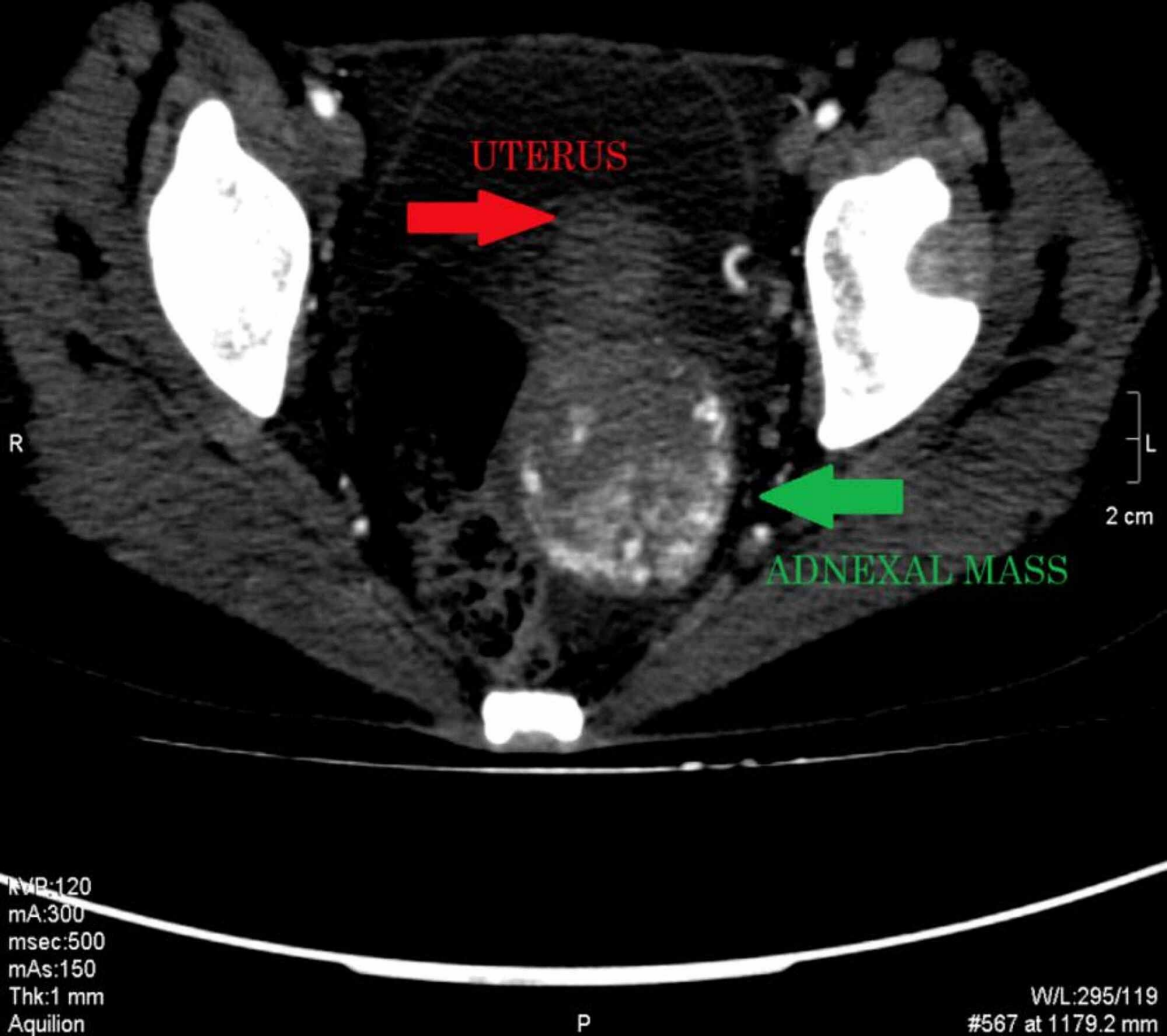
CECT pelvis axial section showing a large highly vascular mass in the left adnexal region extending up to the posterior cul-de-sac displacing the uterus anteriorly CECT: contrast-enhanced computed tomography
